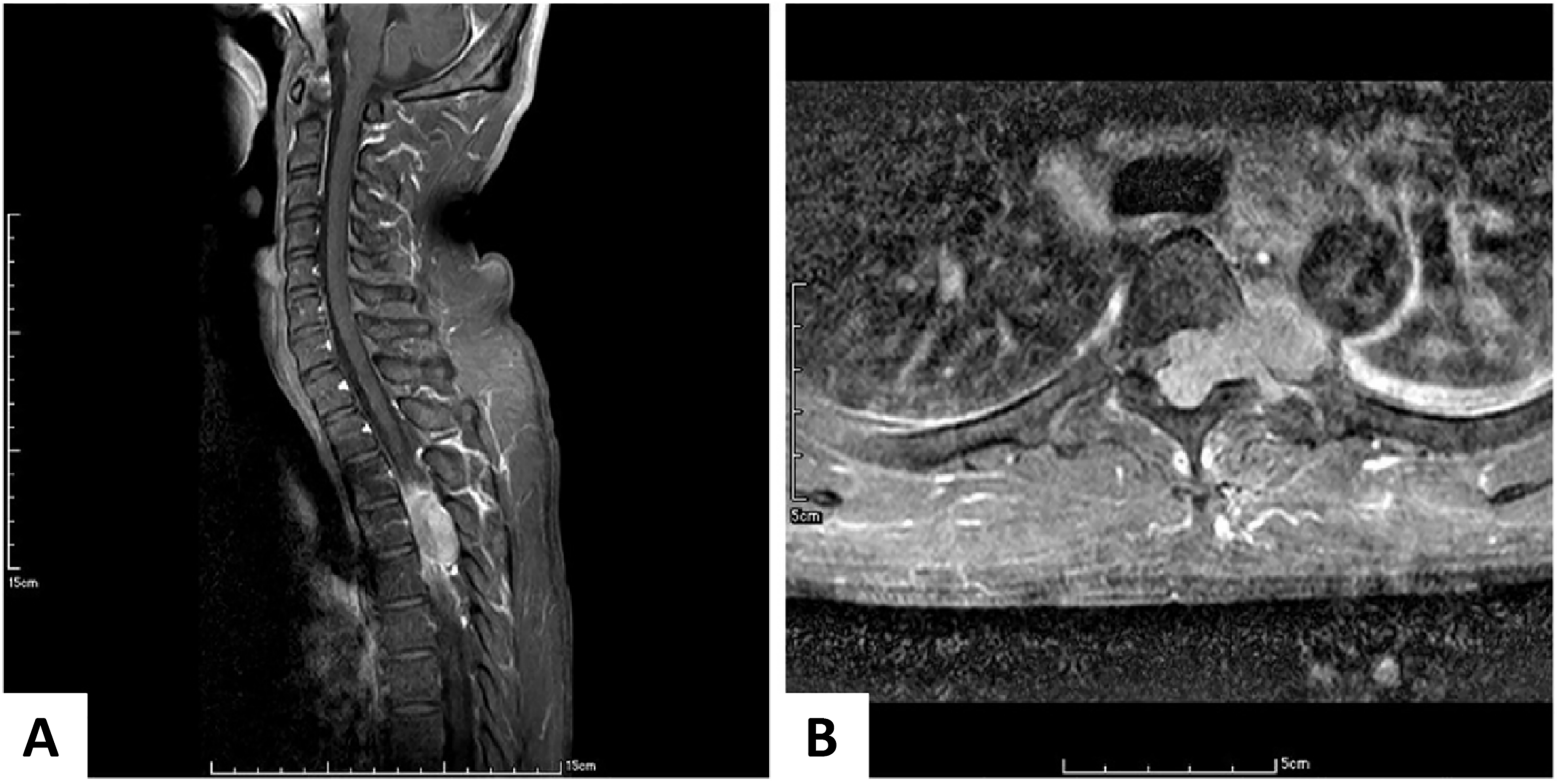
Enhanced Magnetic Resonance Imaging of the Cervicothoracic Spine. (A) In the sagittal view, a large enhancing epidural tumor arising from T4 compresses the spinal cord. (B) In the axial view, this tumor arises from the left neural foramen and pushes the spinal cord to the right. Similarly, the tumor involves the aorta.
